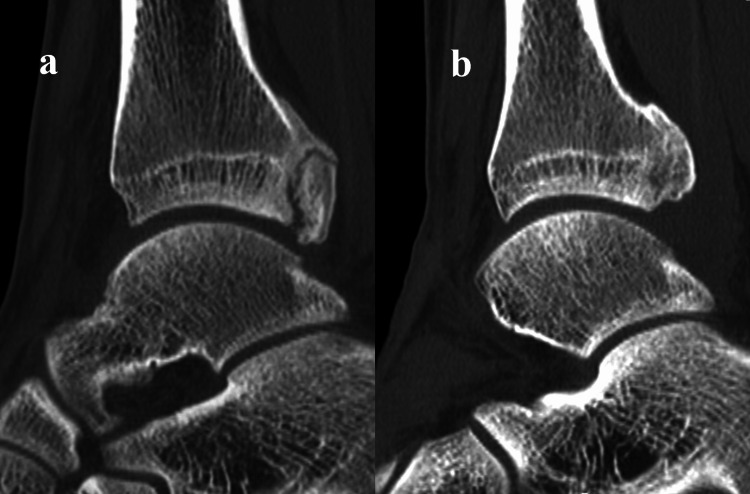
Sagittal reformat of a computed tomography (CT) scan (a) Preoperative image demonstrates nonunion with malformed callus of posterior malleolar fracture. (b) Postoperative image demonstrates union of the residual fragment
